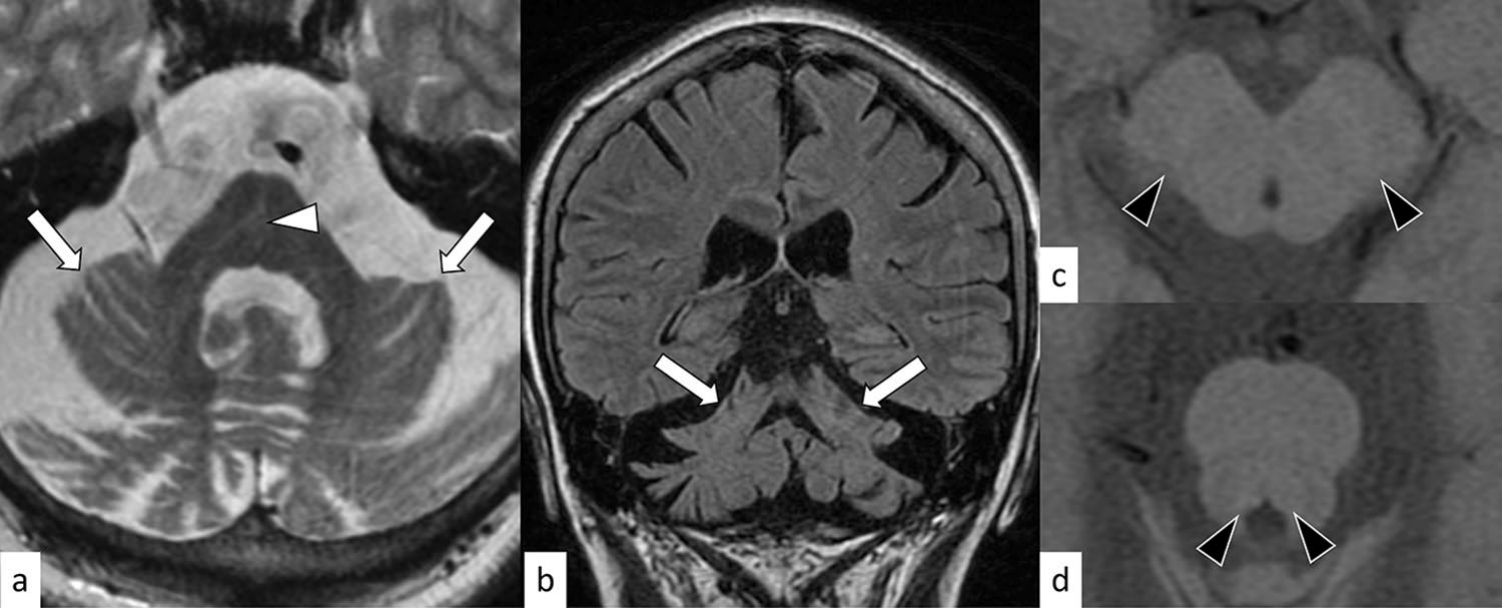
A 33-year-old woman with SCA2. T2-weighted axial image a and FLAIR coronal image b show severe cerebellar atrophy (arrows). The hot cross bun sign is also observed (a, white arrowhead). Decreased melanin deposition in the bilateral substantia nigra c and locus ceruleus d is present on neuromelanin images (black arrowheads)
